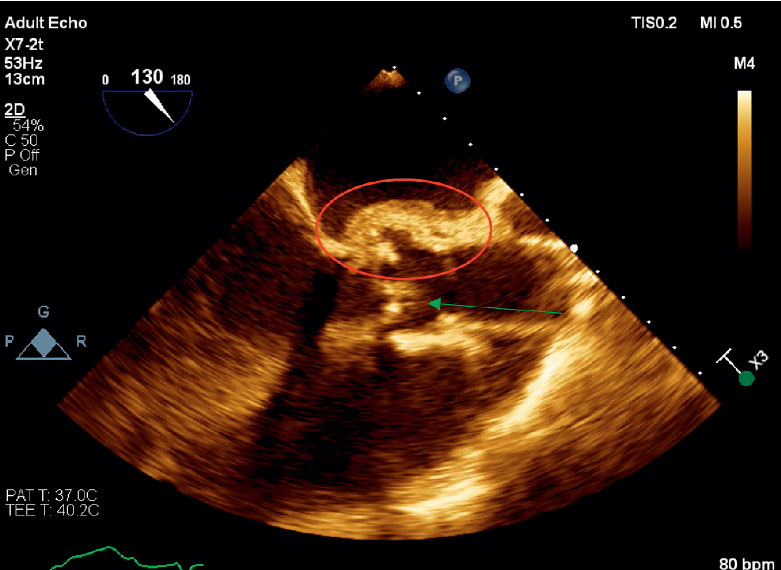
Transesophageal long axis view of the aortic valve. Paravalvular abscess is denoted by the circle. The arrow denotes vegetation on the aortic valve.
